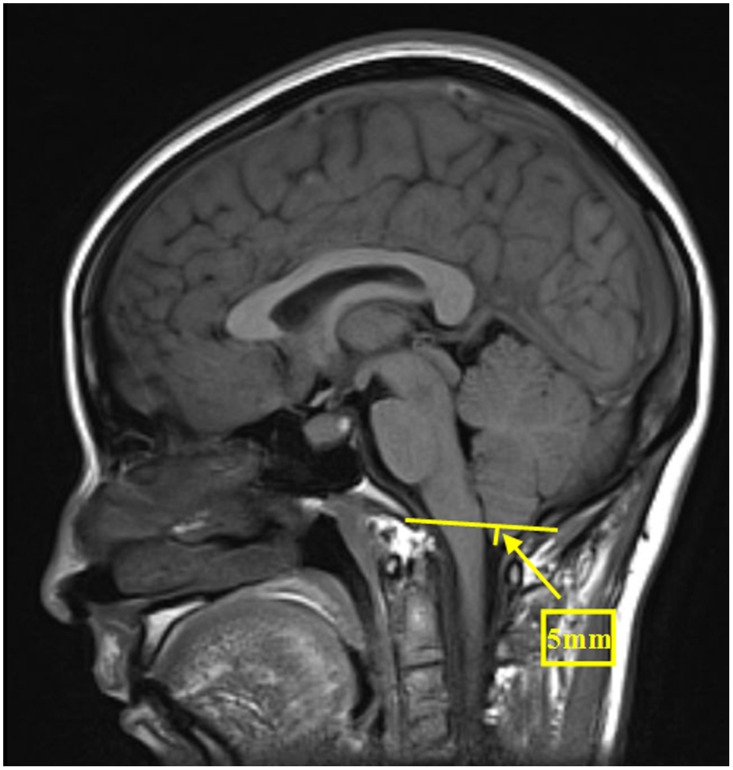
Typical MRI manifestation of brain sagging. Midsagittal T1-weighted MRI shows downward displacement of the cerebellar tonsil by 5 mm (arrow).
